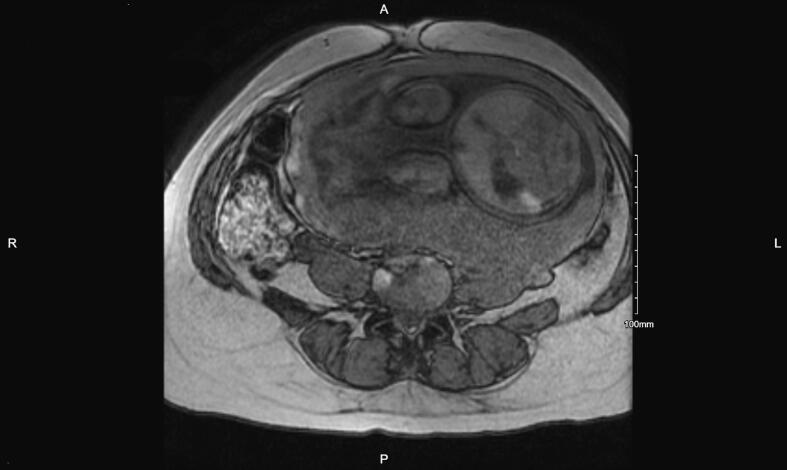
Antepartum MRI abdomen/pelvis showing resolution of right lower quadrant implant after FOLFOX.
